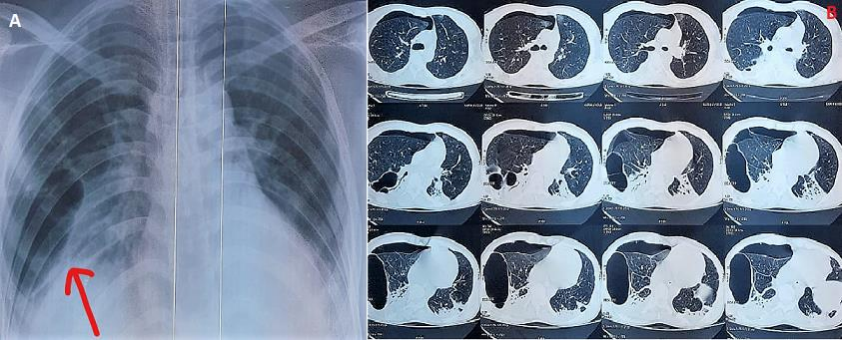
A) chest X-Ray: thin walled cavities; B) chest CT scan: multiple and large air filles cavities in the right lung
